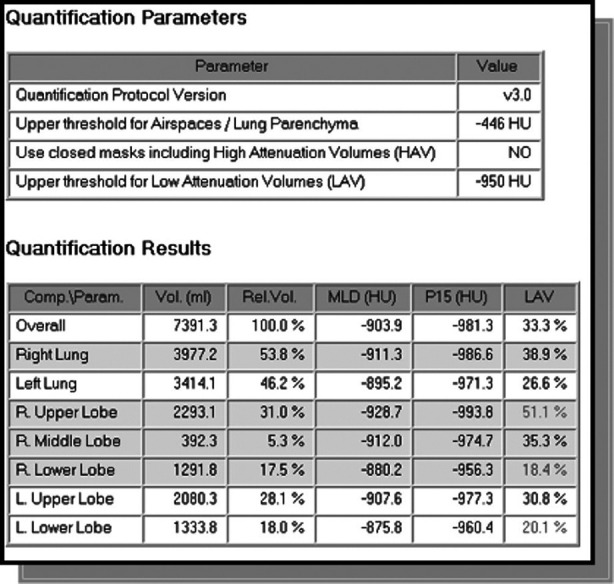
Lobe based functional CT parameters.
